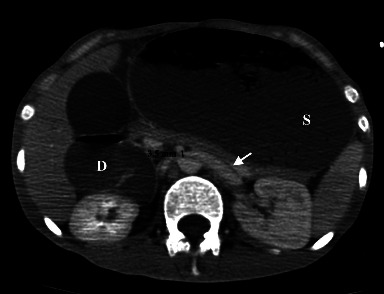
Axial CT scan showing an aortomesenteric distance of 3.5 mm, an extremely distended stomach (S) and duodenum (D), and a dilated left renal vein (white arrow) before the mesenteric clamp.
